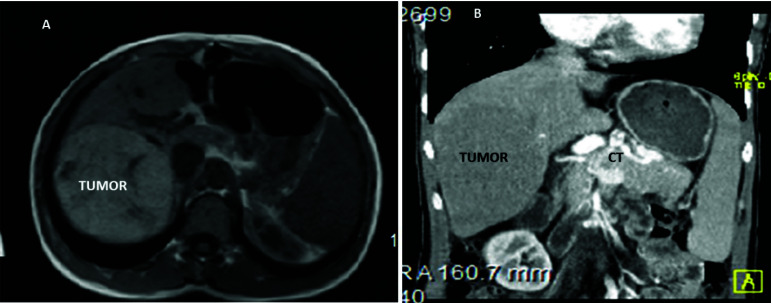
(A, B) Magnetic resonance abdominal image: Right lobe hepatic tumor (hepatocellular adenoma), obliteration and cavernous transformation (CT) of the portal vein, splenomegaly.
